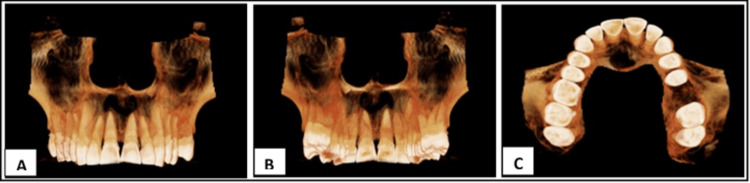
Maxillary CBCT imaging showing radicular cyst involving anterior quadrant: A) buccal view; B) palatal view; C) occlusal view CBCT - cone beam computed tomography
